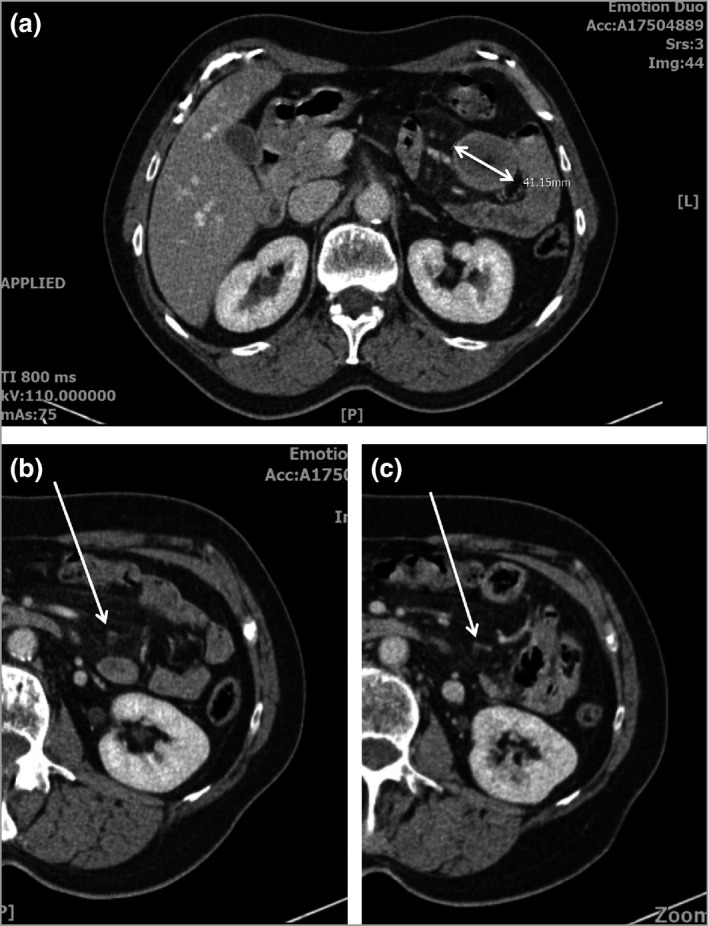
(a) Baseline pretreatment computed tomography (CT) image highlighting a large mesenteric cyst: the double‐headed white arrow indicates a span of 41·15 mm width. (b) A small mesenteric cyst detected at baseline CT before treatment, indicated with a white arrow. (c) Two months after commencing vismodegib treatment, the same mesenteric cyst is smaller.
